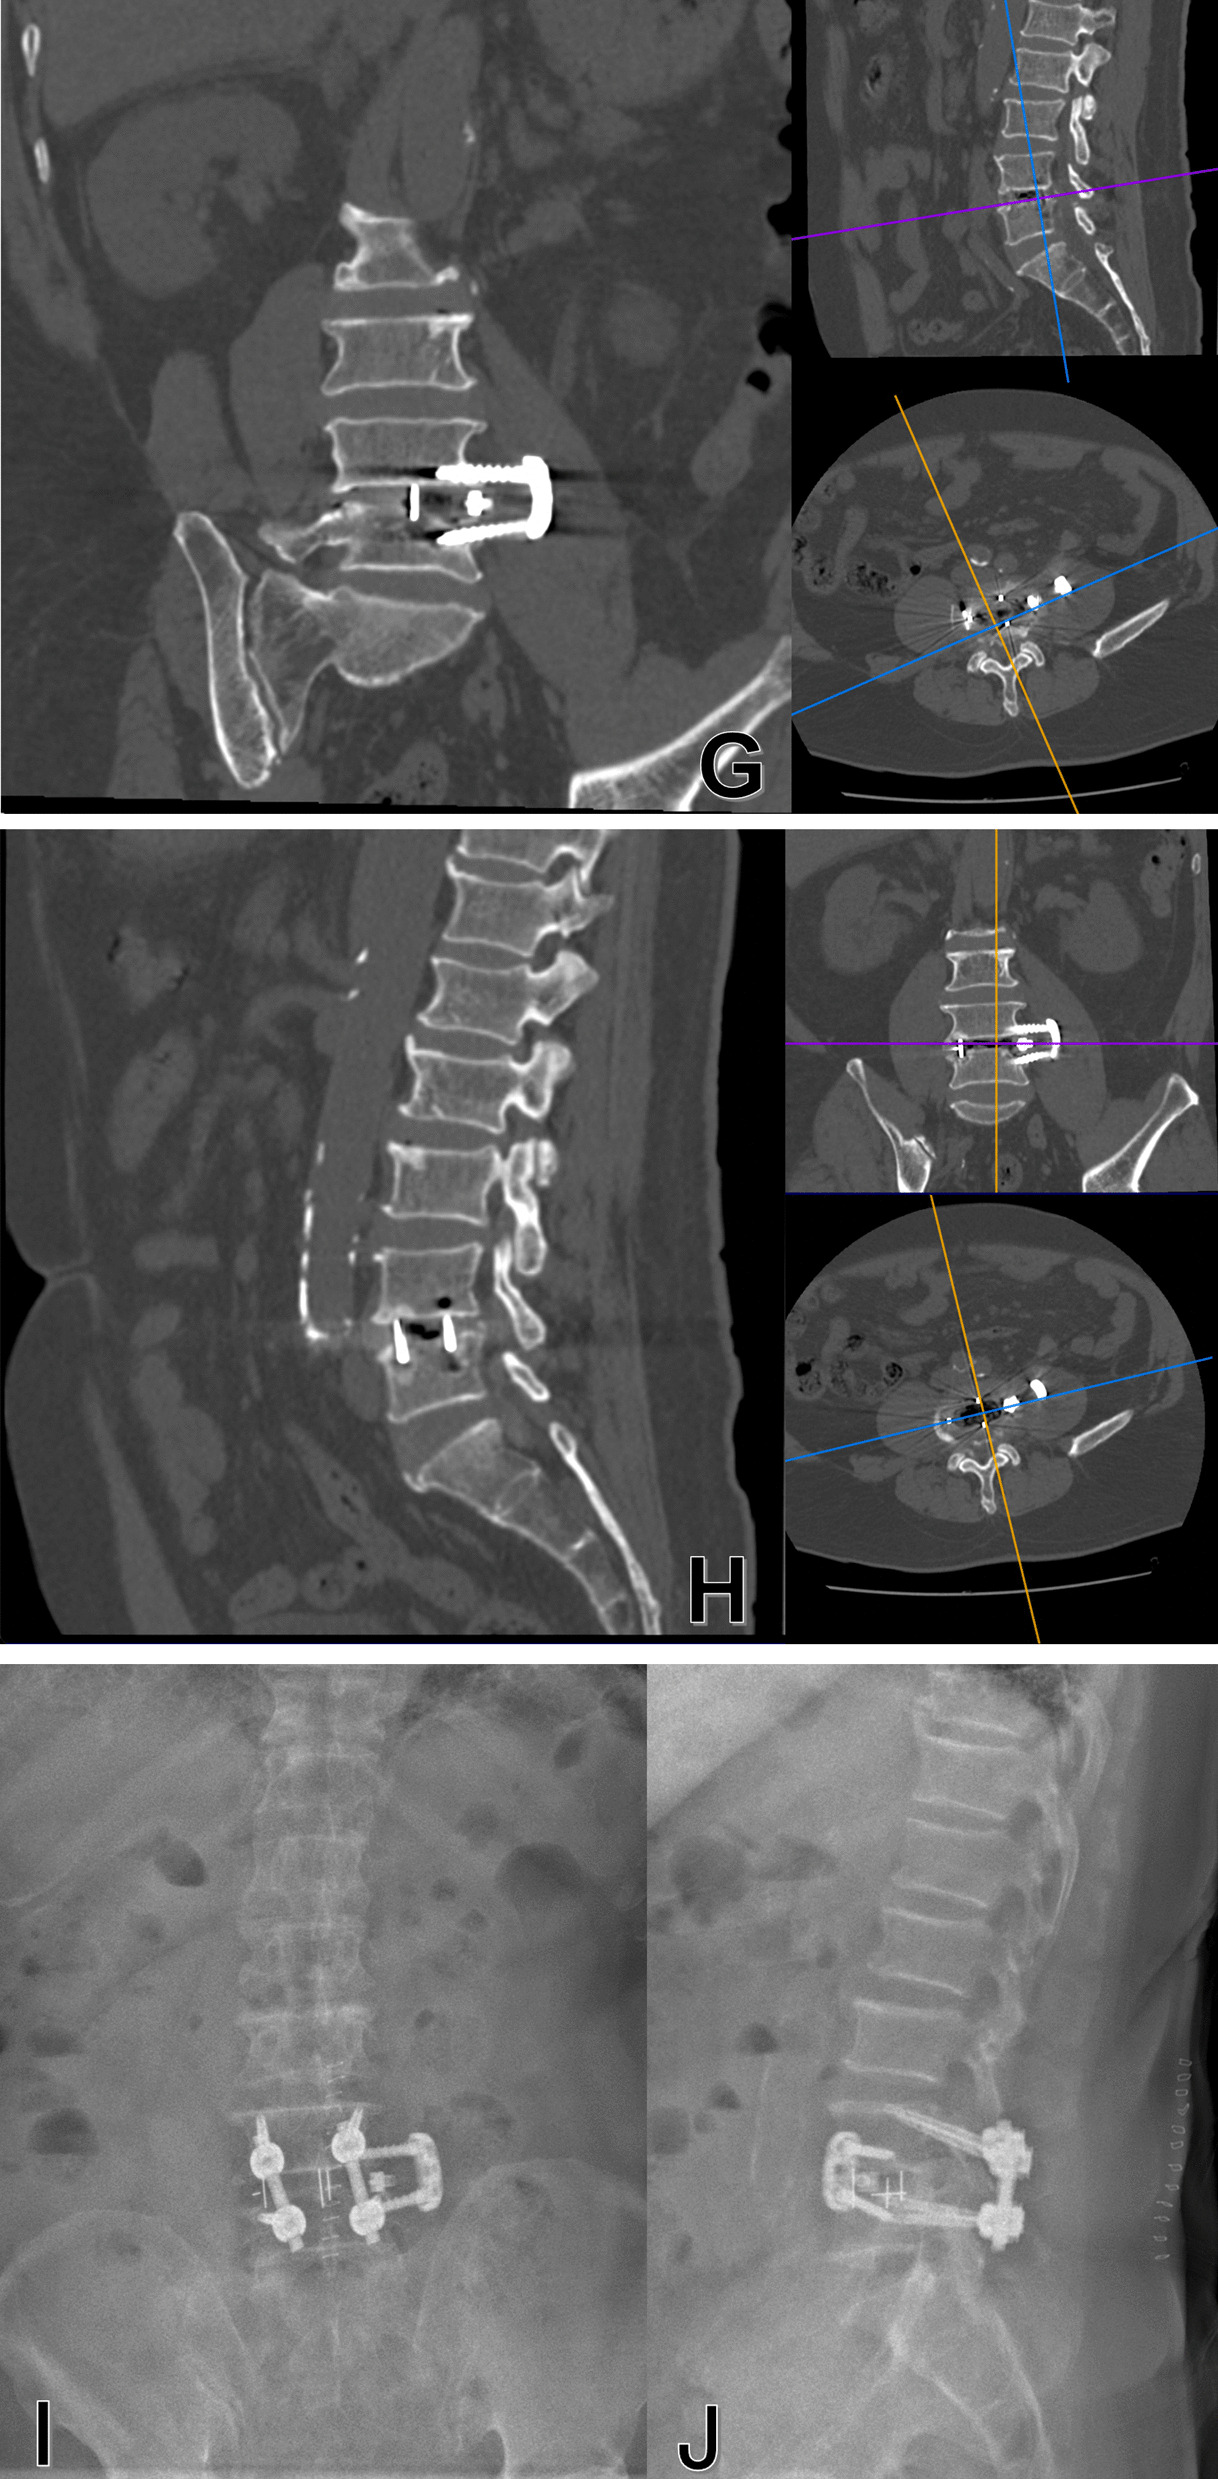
Images obtained in a 77-year-old man who presented with back pain and neurogenic intermittent claudication (BMI: 29.7 kg/m^2^; BMD: 114.1 mg/cm^3^). a and b Preoperative magnetic resonance imaging revealed moderate stenosis at L_4–5_. c and d Postoperative anteroposterior and lateral radiographs revealed the satisfactory position of hardware. The angle between screws was 17.8° in the anteroposterior radiograph. e and f Anteroposterior and lateral radiographs at 7 weeks after the initial surgery revealed cage subsidence and the migration of lateral plate and screws. The angle between screws was 7.9° in the anteroposterior radiograph. g Computed tomography coronal reconstruction confirmed the migration of lateral plate and screws. h Computed tomography sagittal reconstruction confirmed cage subsidence. i and j Anteroposterior and lateral radiographs after revision surgery with cortical bone trajectory screw placement in midline lumbar fusion (MIDLF) and left L4 laminotomy
